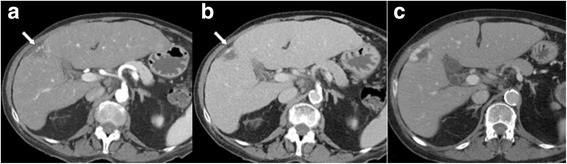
LR-2 a Arterial phase MDCT shows a subcapsular lesion in the right lobe with capsular retraction and peripheral nodular enhancement, with b progressive centripetal enhancement in the venous phase. Diagnosis is probable haemangioma (LR-2). c A previous CT scan, performed 9 years earlier, could be obtained, which confirmed the diagnosis of haemangioma
